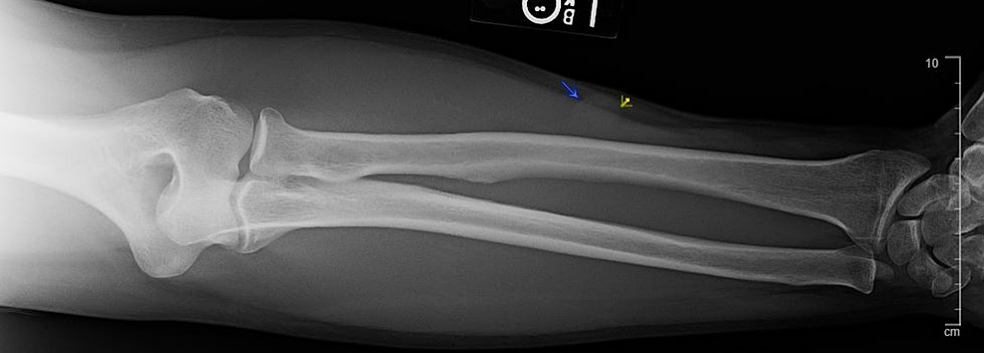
Plain radiograph image of left forearm shows 1.6 cm ovoid density within the lateral soft tissues.
